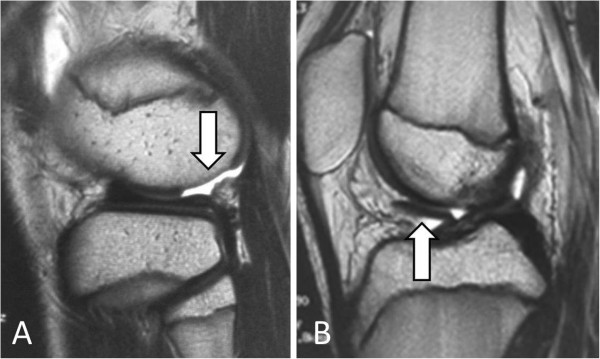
T2-weighed sagittal magnetic resonance images at the initial visit. (A) A full-thickness chondral defect in the weight-bearing portion of the lateral femoral condyle (white arrow). (B) A large chondral fragment in the anterior joint space (white arrow).
